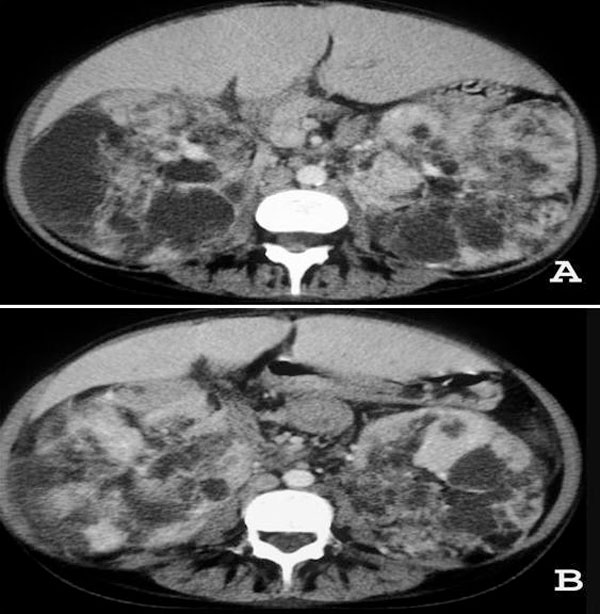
CT of the upper abdominal region demonstrates bilateral giant renal masses consisting predominantly of fat tissue. The density measurement of the hypodense content ranged from −15 to −148 Hounsfield units.
